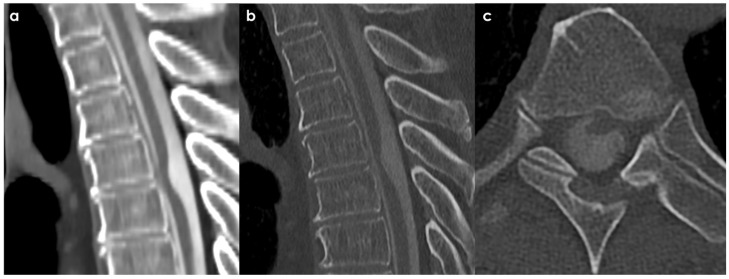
Detail of the sagittal (a,b) and axial CT Myelography (c) with the magnified finding of spinal cord herniation. (b,c) are in a soft tissue window.
